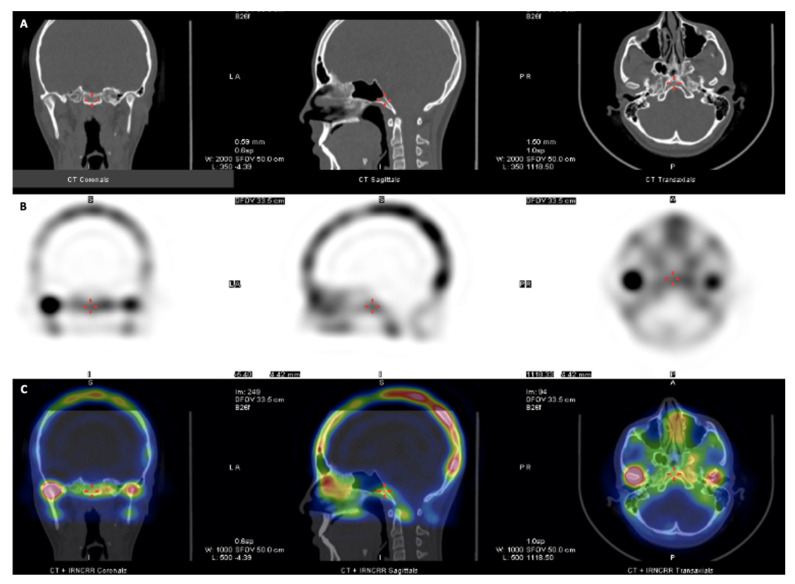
(A) Computed tomography (CT) images: coronal, sagittal, and axial sections. (B) Single photon emission CT (SPECT) images: coronal, sagittal, and axial sections. (C) Fused functional images (SPECT and CT) in a patient with right side active unilateral condylar hyperplasia (UCH).
